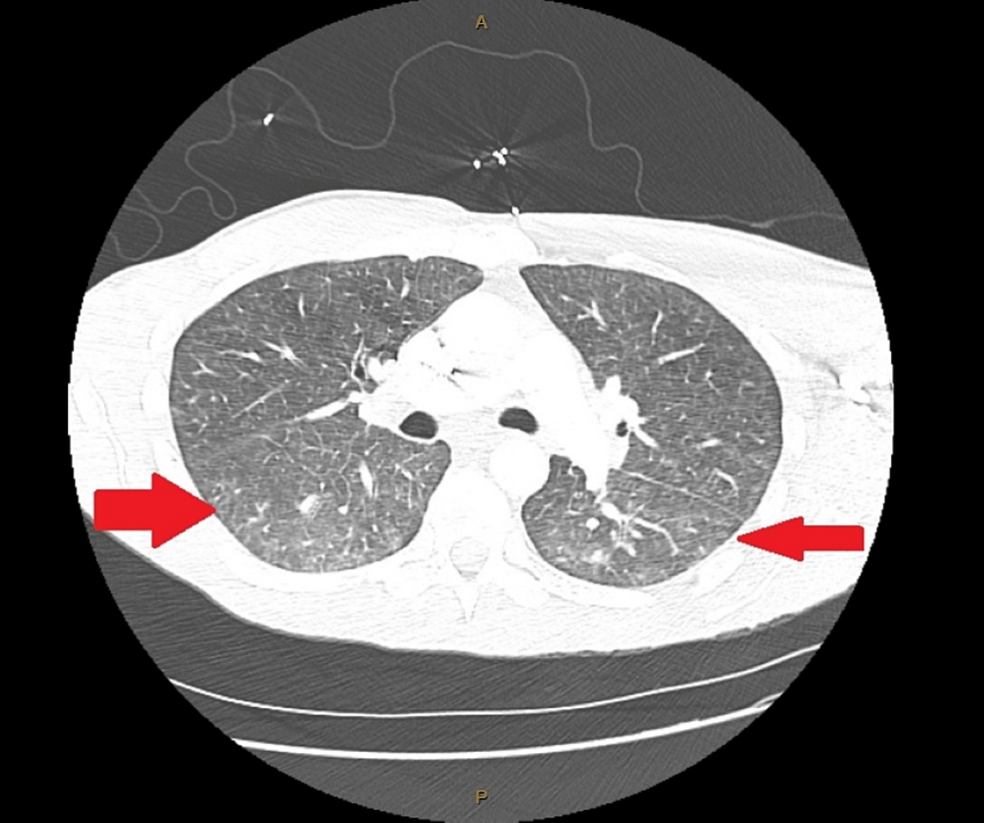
CT chest showing ground-glass opacities and pulmonary nodules CT: computed tomography
